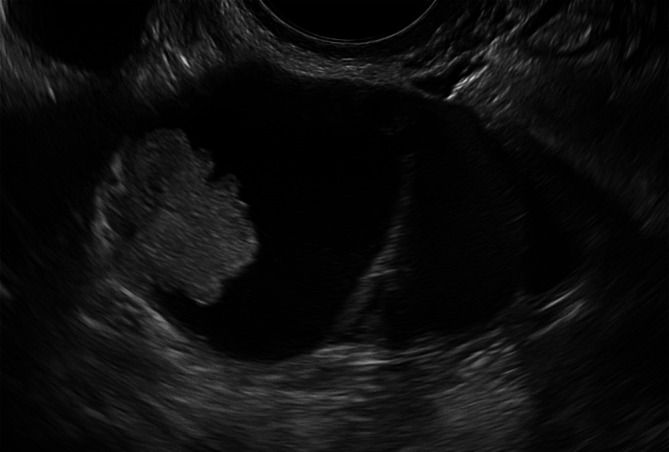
Transvaginal gray‐scale ultrasound image, in axial view, of a seromucinous borderline ovarian tumor (lateral) with adjacent endometrioma (medial) of the right ovary.
